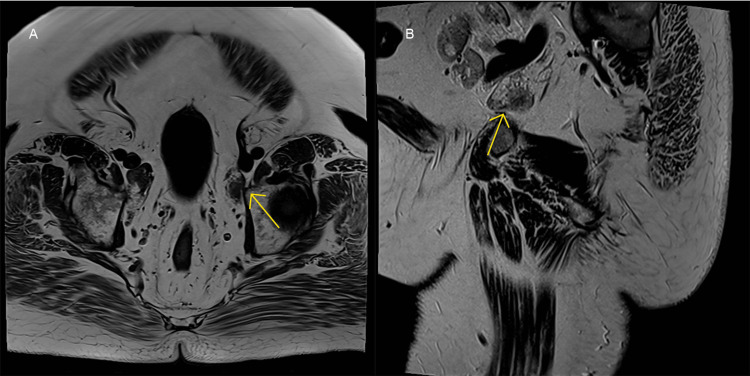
T1-weighted MRI of the pelvic lymph nodes. (A) Axial T1-weighted and (B) coronal T1-weighted slices demonstrating an enlarged, 3.6cm left sided lymph node within the external iliac region (yellow arrows).
